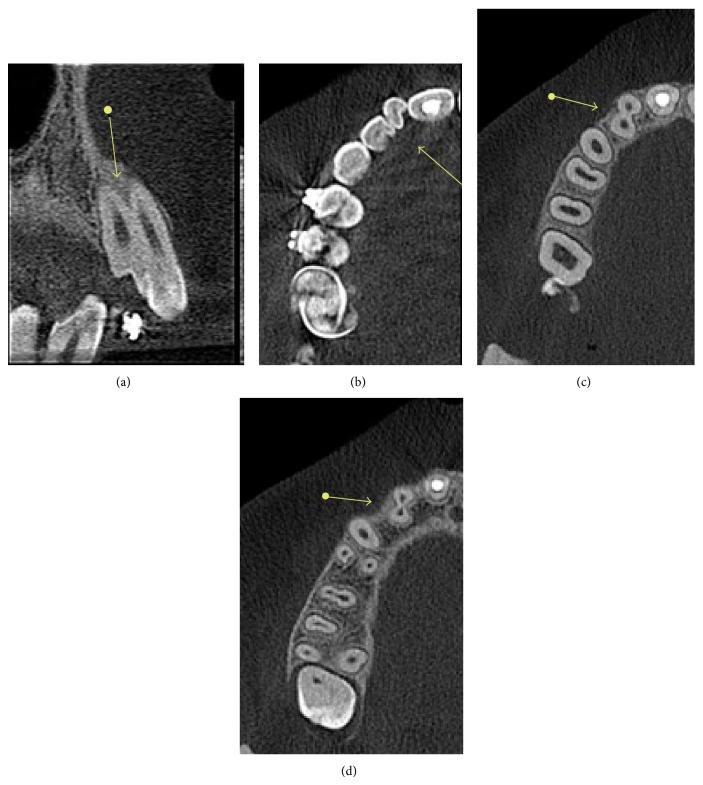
(a) Cross section of the cone beam computed tomography (CBCT) reveals the union of the right maxillary lateral incisor and the supernumerary tooth at the dentin level and shows the extent and limits of the anomaly, suggesting dental fusion. Axial sections of the CBCT show signs of fusion in the (b) cervical third, (c) middle third, and (d) apical third of the root.
